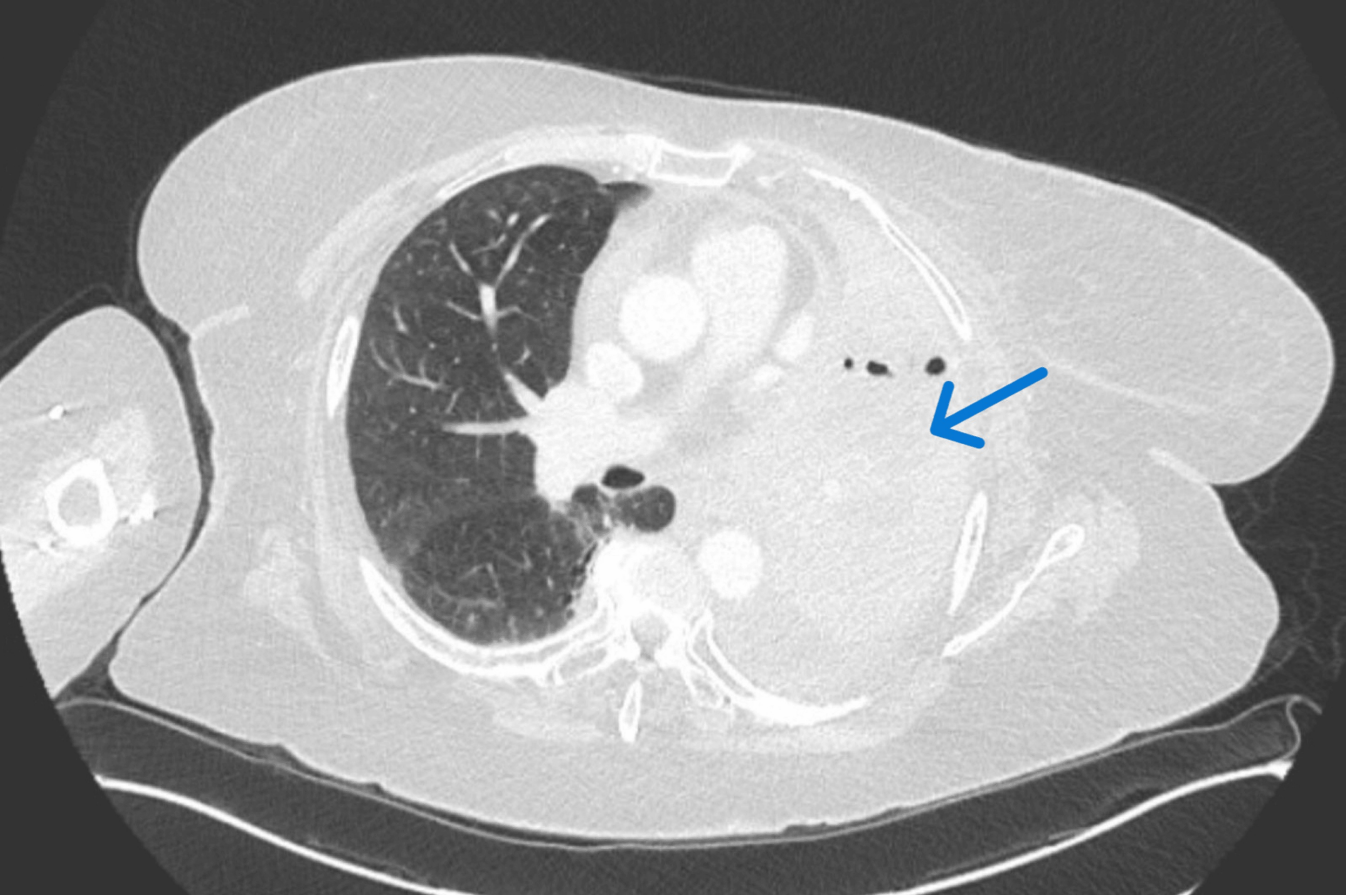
CT Chest After Three weeks of High-Dose Prednisone Treatment Stable left bronchus occlusion (blue arrow) with no new foci. The resolution of ground-glass opacities suggests effective treatment and resolution of checkpoint inhibitor pneumonitis with high-dose steroids and IVIG.
